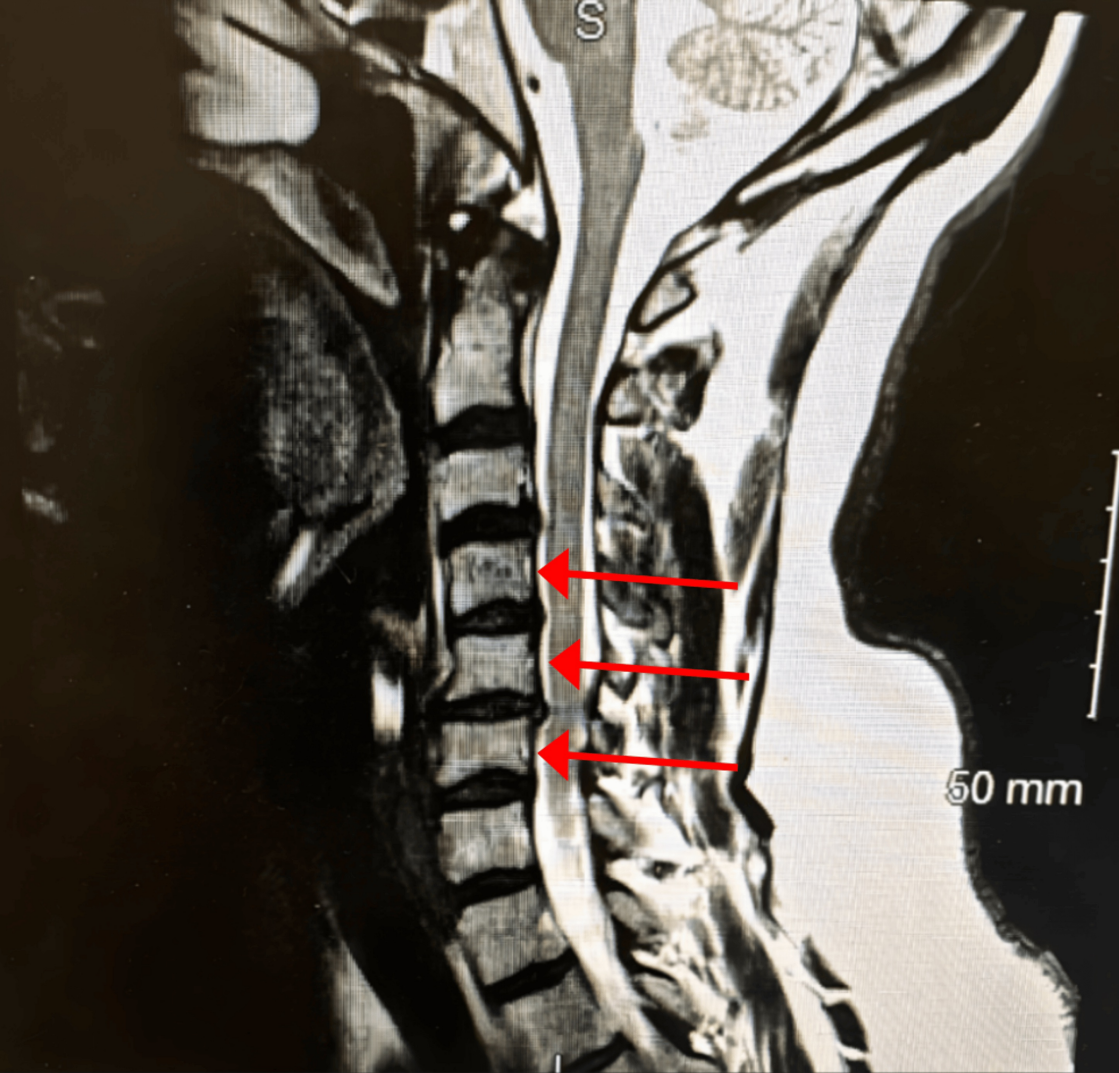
Sagittal MRI of the cervical spine demonstrating multilevel degenerative changes (red arrows) S: Superior
